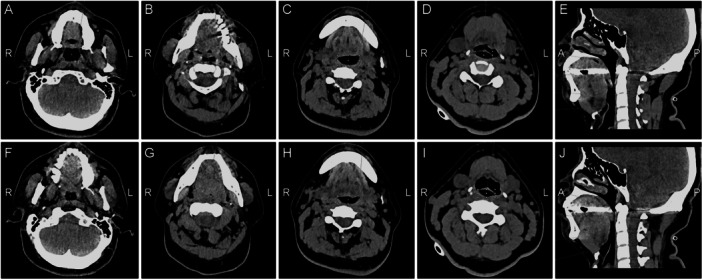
Preoperative upper airway computed tomography images during quiet breathing and deep inspiration. (A–E) Axial images during quiet breathing: (A) nasopharynx; (B) velopharynx; (C) retroglossal region; (D) retroepiglottic region; and (E) sagittal segmentation. (F–J) Axial images during deep inspiration: (F) nasopharynx; (G) velopharynx; (H) retroglossal region; (I) retroepiglottic region; and (J) sagittal segmentation.
